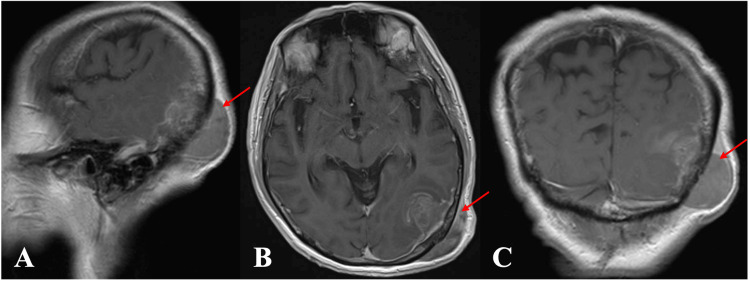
(A) Sagittal, (B) axial, and (C) coronal preoperative images of MRI brain (T1-weighted with contrast) showing right parasagittal tumor (red arrow) MRI: magnetic resonance imaging
